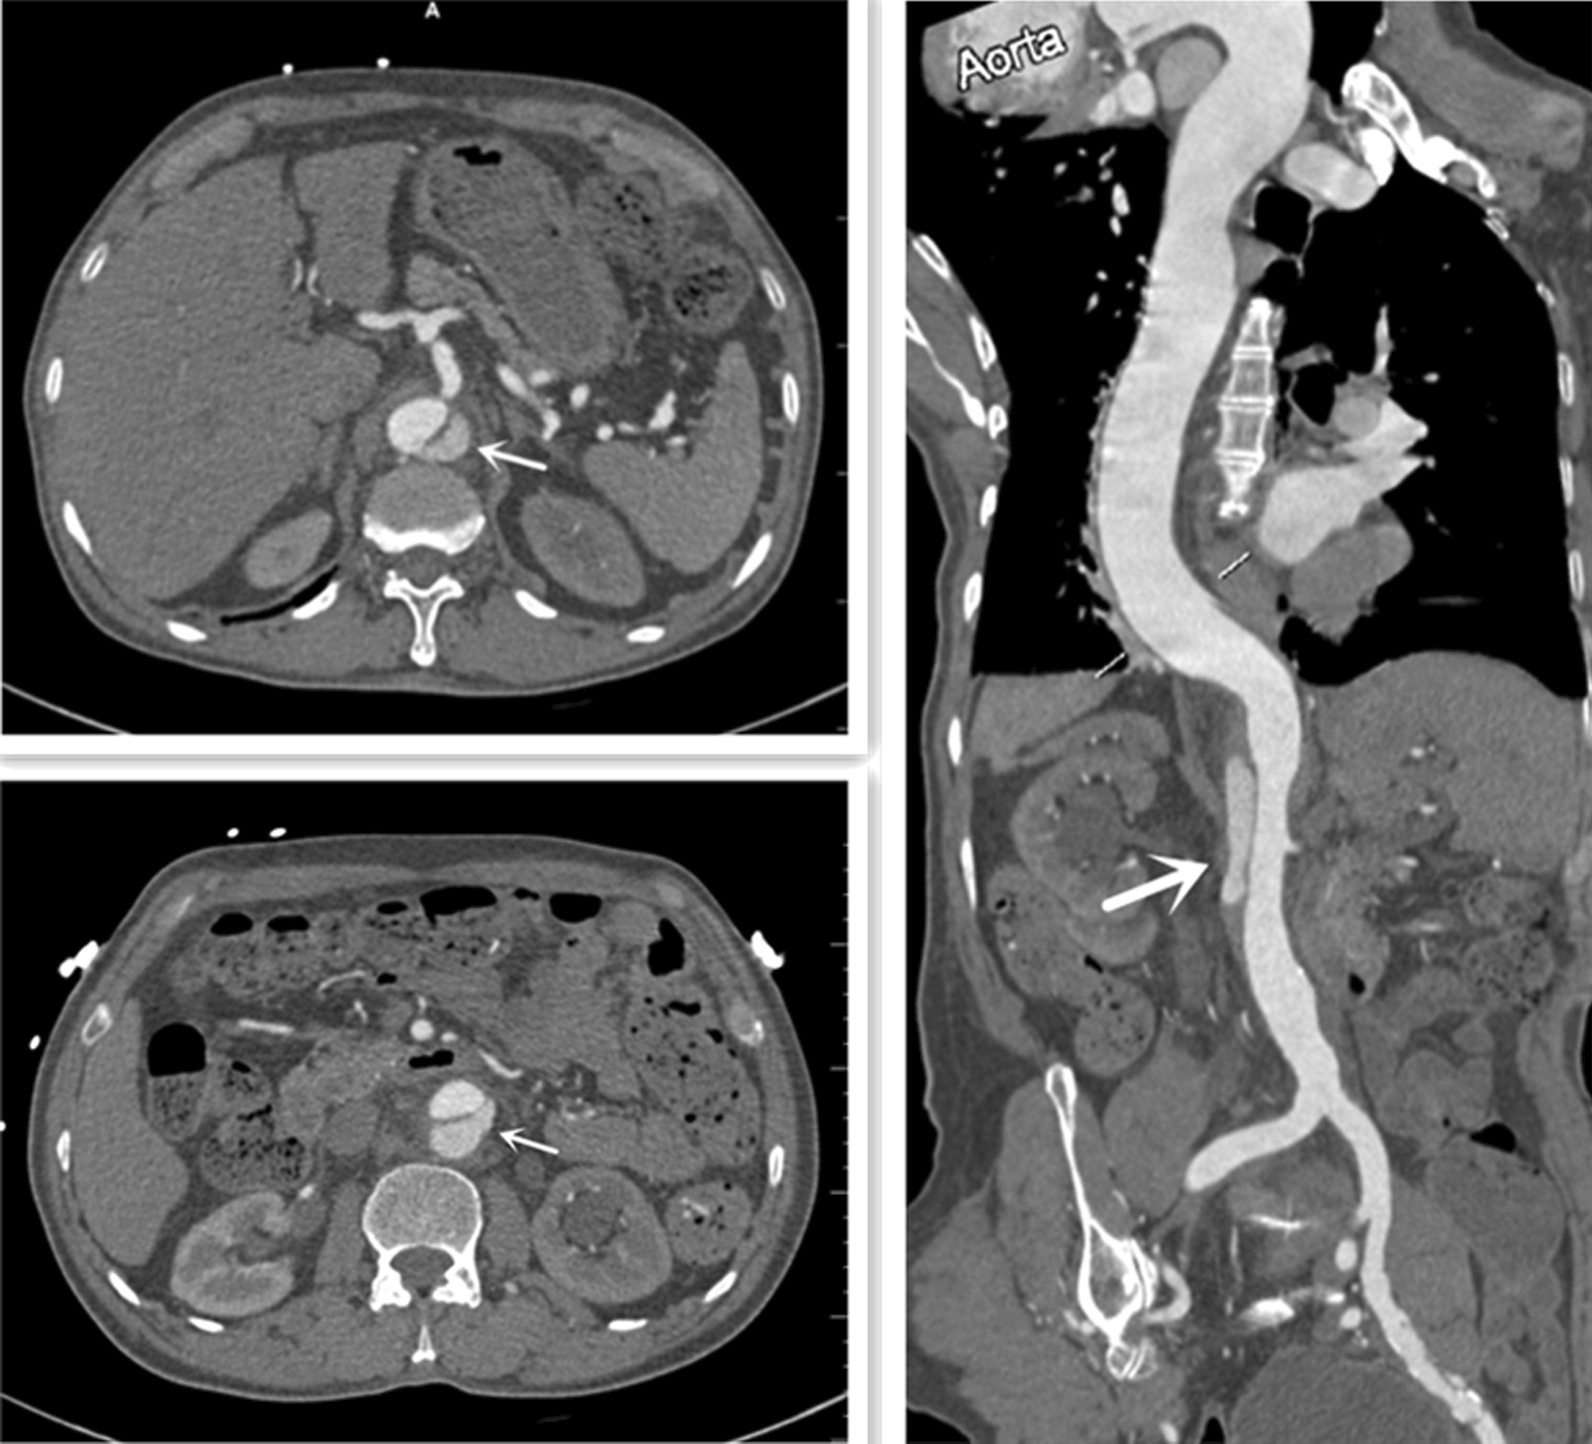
Initial the thoracoabdominal aorta computed tomographic angiography showing penetrating atherosclerotic ulcer in the descending aorta. The white arrow refers to the false lumen at the level of abdominal aorta and Mesentery artery before operation
